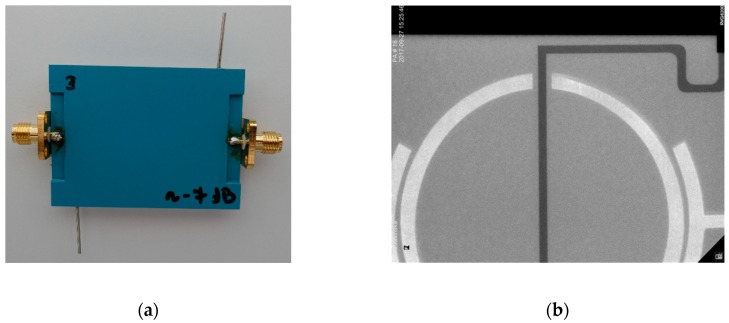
The resonator-based sensor: (a) photo, (b) X-ray image for a resonator and microchannel alignment.
